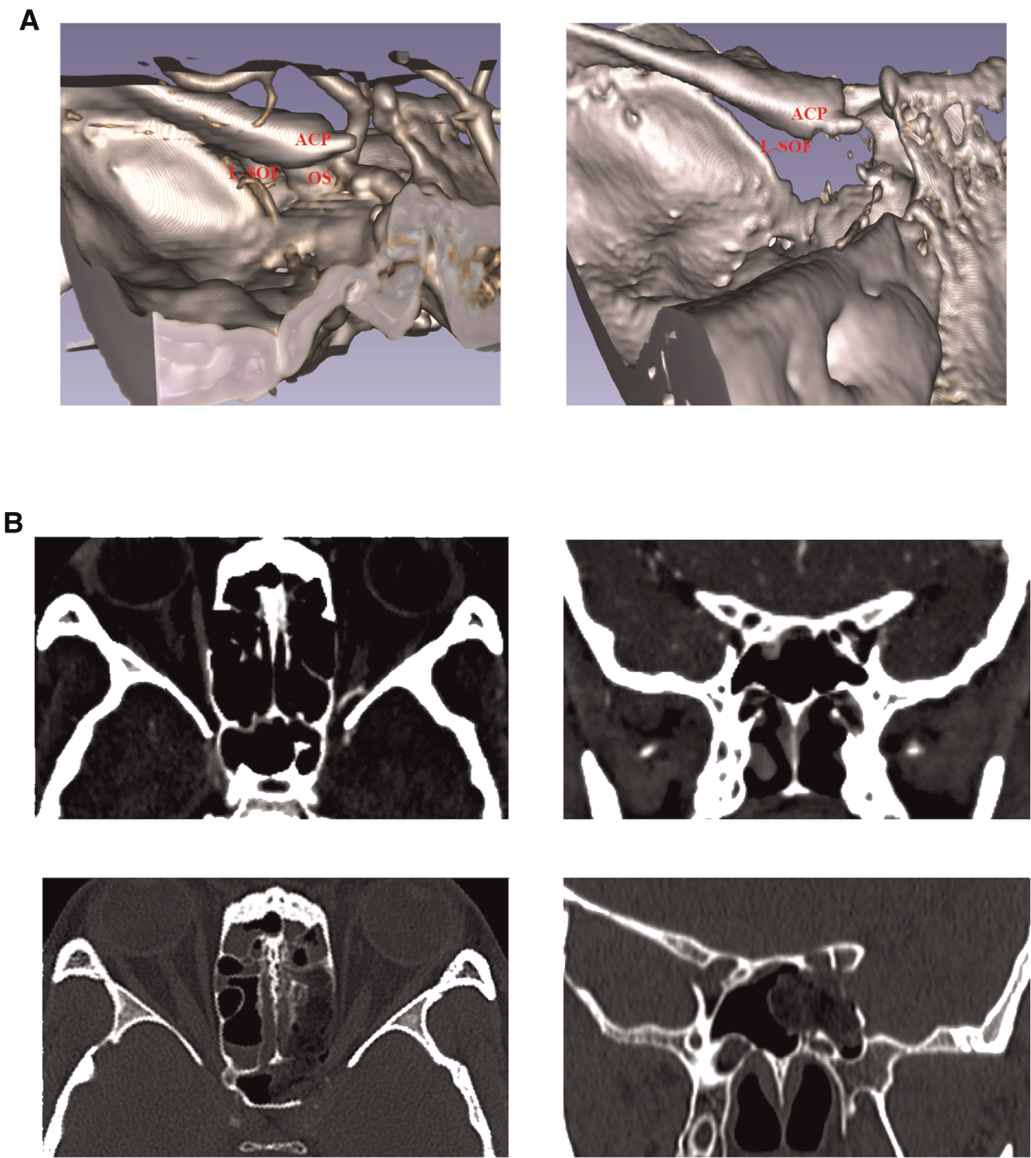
Comparison of preoperative and postoperative imaging data. (A) Observation of superior orbital fissure from the upper left of the skull base. Preoperative and postoperative 3D reconstruction images are shown on the left and right, respectively. It can be seen that the optic strut and part of the body of the sphenoid bone are resected. (B) Postoperative thin-slice CT reexamination. Left: Comparison of axial CT. Right: Comparison of coronal CT. Top: Preoperative images. Bottom: Postoperative images.
